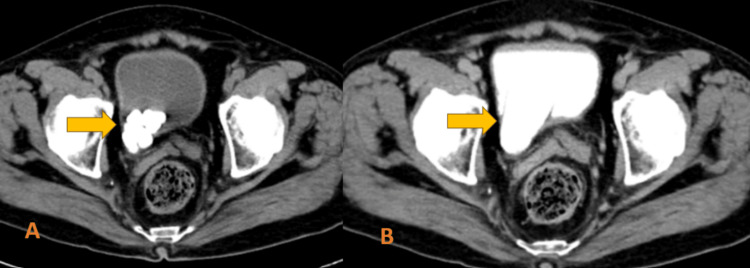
Axial CT sections of the lower abdomen non-contrast (A); and delayed phase of urography (B) showing multiple hyperdense calculi in the vesico-ureteric junction on the right side with invagination of the distal ureter into the urinary bladder
